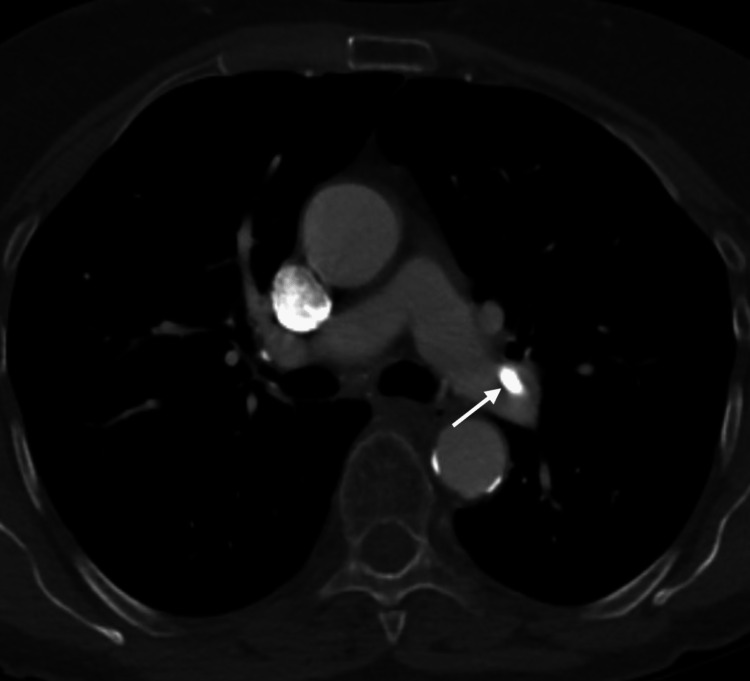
Computed tomography pulmonary angiogram High-density material representing a pulmonary cement embolism in the left main pulmonary artery is denoted by the white arrow.
